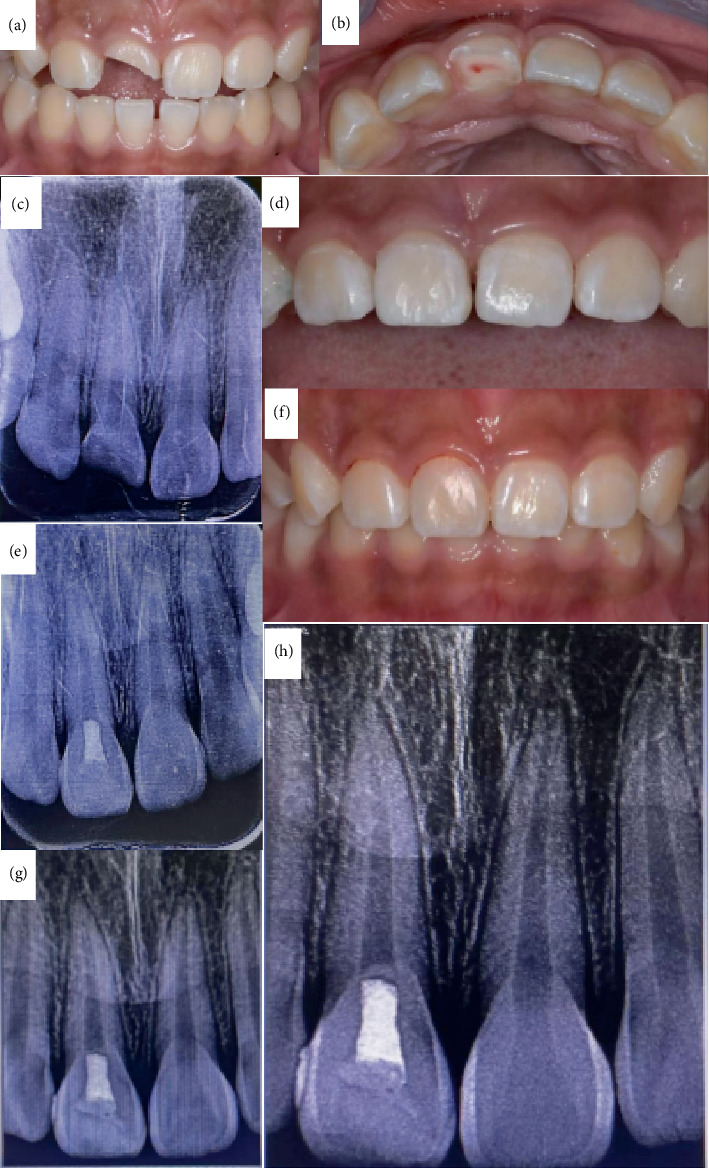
Patient treatment process. (a) Initial frontal view shows fracture of the crown of Tooth 11. (b) Initial incisal view shows exposure of the pulp hole at the fracture end of Tooth 11. (c) Initial periapical radiograph shows fracture of the crown of Tooth 11, root development at Nolla Stage 9, no image of root fracture, continuous periodontal ligament without widening. (d) Immediately after pulpotomy and crown reattachment surgery for Tooth 11. (e) Periapical radiograph immediately after surgery for Tooth 11. (f) Frontal view at 1-month follow-up after crown reattachment surgery. (g) Periapical radiograph at 1-month follow-up after crown reattachment surgery shows slight calcification bridge image change at the root canal orifice of Tooth 11, with no abnormalities seen in the periapical area. (h) Periapical radiograph at 6-month follow-up after crown reattachment surgery shows significant calcification bridge image change at the root canal orifice of Tooth 11, with no abnormalities seen in the periapical area.
